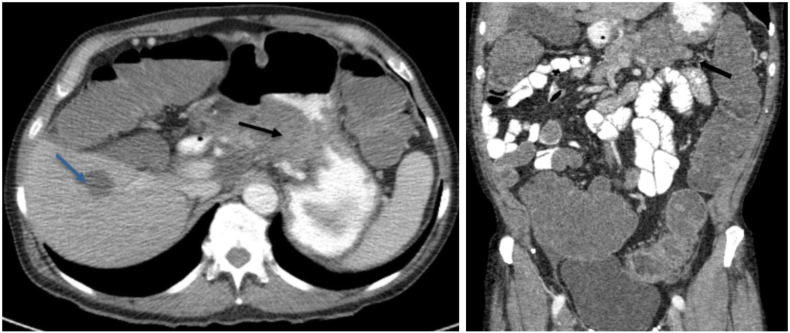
Axial (left) and coronal (right) CT scan - large pancreatic body mass invading the posterior wall of the stomach (black arrow) and hepatic metastatic lesion (blue Arrow).
